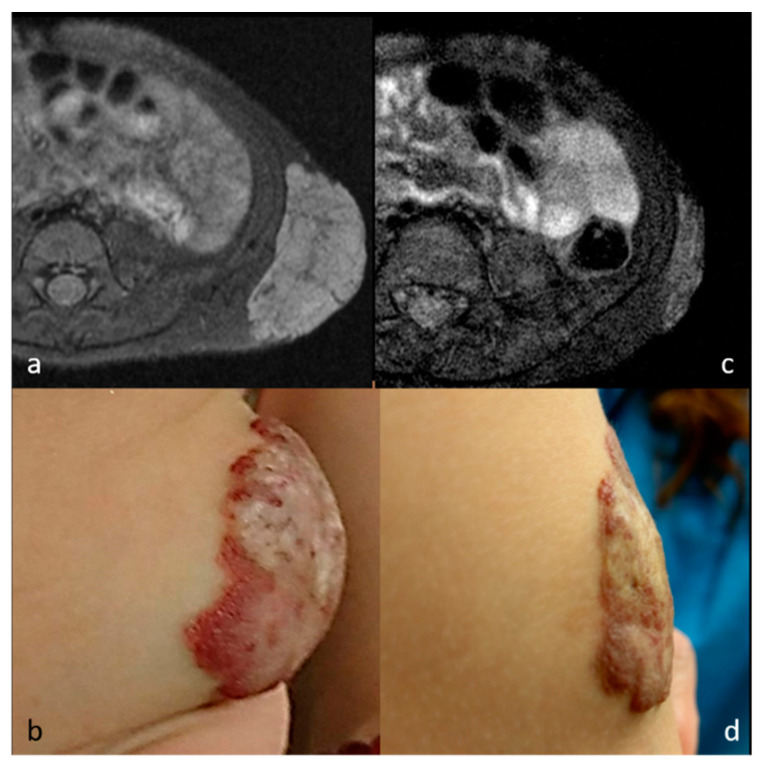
MR images of patient 5 before (a) and after (c) RFA show significant shrinkage of the congenital hemangioma in the left abdominal wall. Photographs before (b) and after (d) RFA show the cutaneous and subcutaneous lobular, reddish bulging lesion marked flattened and decolorized into pinkish color (cosmetic scale 4 → 3).
